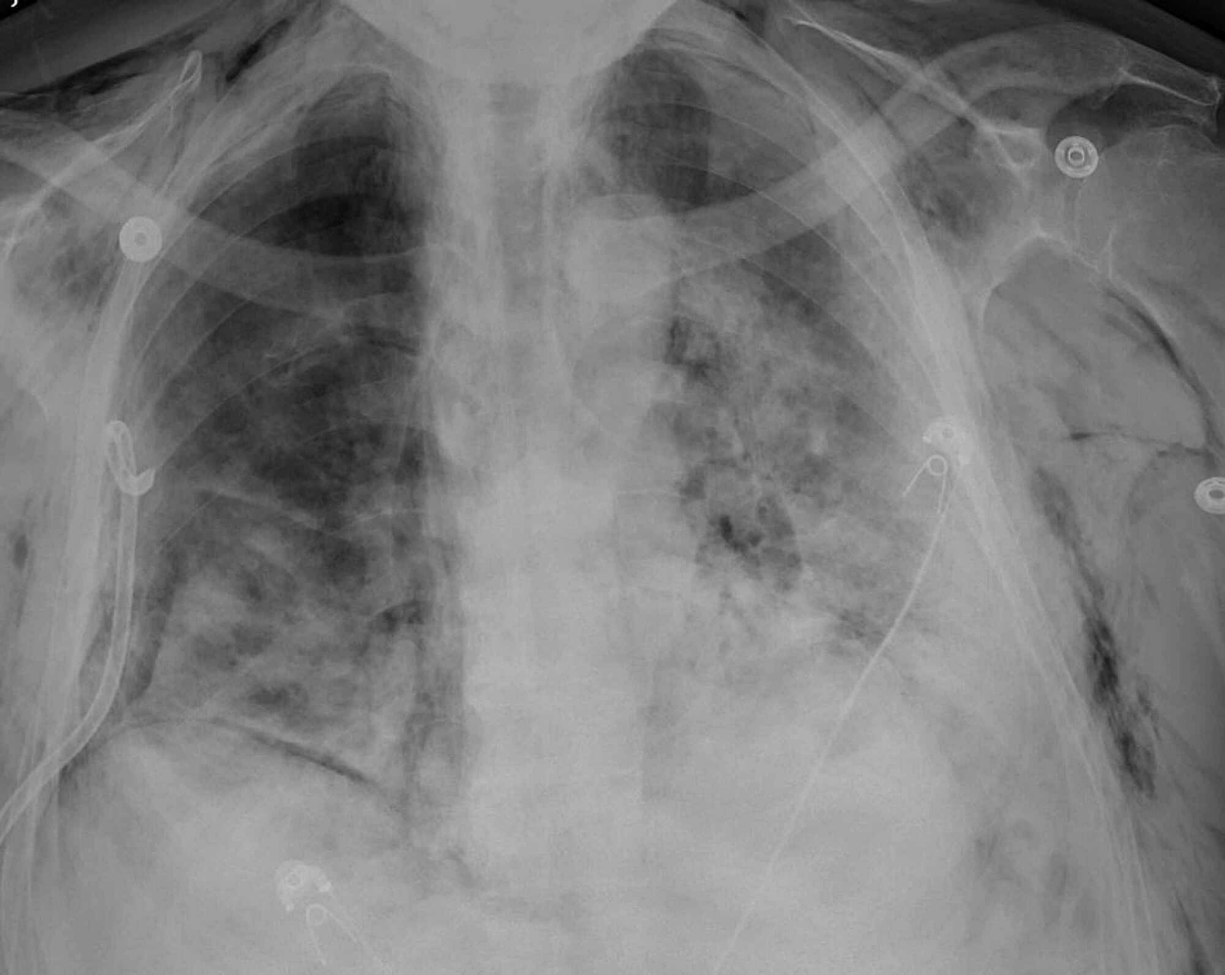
Chest X-ray shows significant improvement of right-sided pneumothorax s/p right tube thoracostomy
